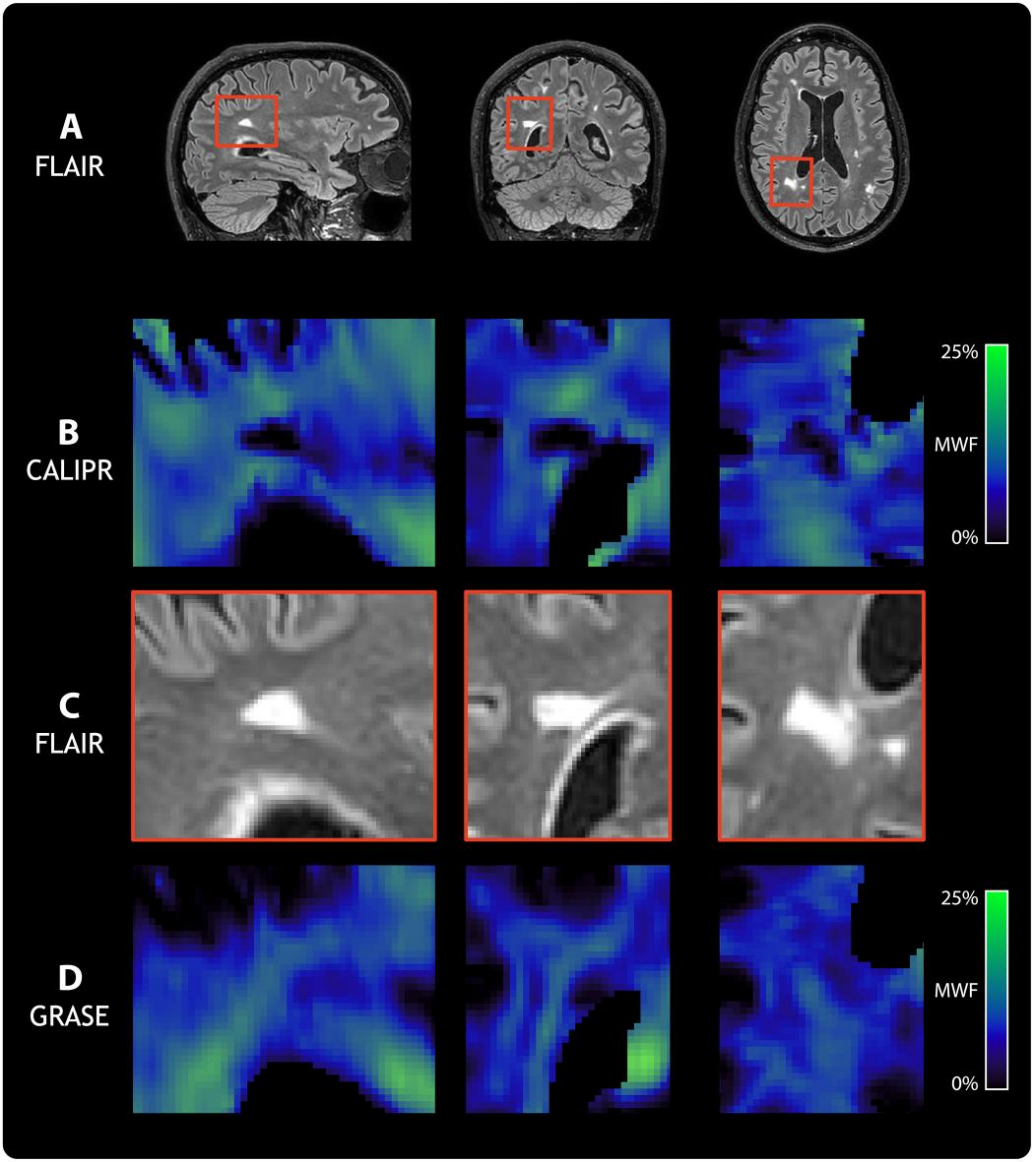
Comparison of MWF sensitivity to demyelinating disease pathology. From left to right, columns show sagittal, coronal, and axial orientation views, respectively, for a subject living with relapsing-remitting multiple sclerosis (male, age 60 years, expanded disability status scale of 2.0, and disease duration of 12 years). (A) Full slices of a FLAIR anatomical image are shown with red boxes highlighting a region centered around a particularly large FLAIR hyperintense lesion. Close-up views of this region are shown in (B) for MWF from our proposed CALIPR MWI approach, in (C) for FLAIR, and in (D) for MWF from a commonly used GRASE MWI approach. All images and maps were aligned in the subject’s T_1_-weighted image space to ensure that the exact same region could be compared.
